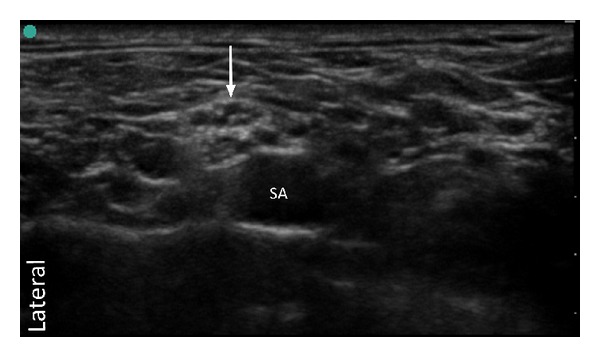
Transverse ultrasound image of supraclavicular brachial plexus. Arrow points toward the divisions of the brachial plexus. The location of the brachial plexus is posterolateral to the subclavian artery (SA).
